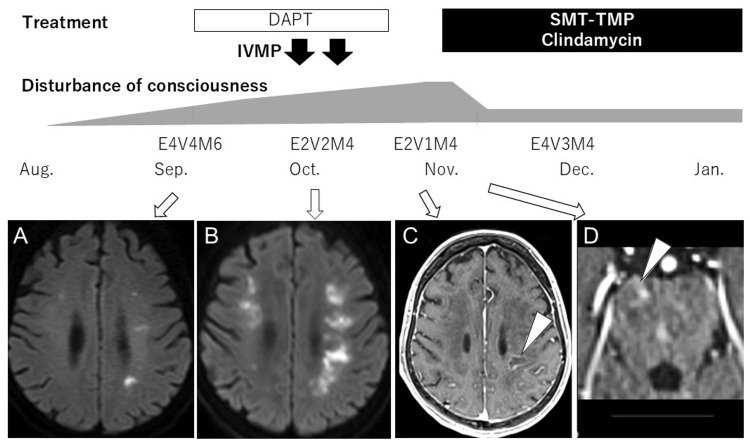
The patient's clinical course and MRI findings In August, the patient developed fatigue and loss of appetite. In September, she presented with a fever, and her level of consciousness declined to a Glasgow Coma Scale (GCS) score of E4V4M6. Brain MRI revealed multiple lesions in the deep white matter of the middle cerebral artery territory (A). The lesions were suspected to be multiple cerebral infarcts, and dual antiplatelet therapy (DAPT) was initiated; however, her level of consciousness continued to deteriorate. Subsequently, mass-like lesions with ring-shaped gadolinium enhancement appeared (arrowheads) in the left parietal lobe (C) and pons (D), raising suspicion for toxoplasmic encephalitis. Treatment with a combination of sulfamethoxazole (SMX)-trimethoprim (TMP) and clindamycin was initiated, leading to an improvement in her consciousness level to a GCS score of E4V3M4. As her condition remained stable, she was transferred to a rehabilitation hospital in January of the following year. (A) and (B): Diffusion-weighted images; (C) and (D): Gadolinium-enhanced T1-weighted images with fat suppression. Aug: August 2024; IVMP: intravenous pulse methylprednisolone
